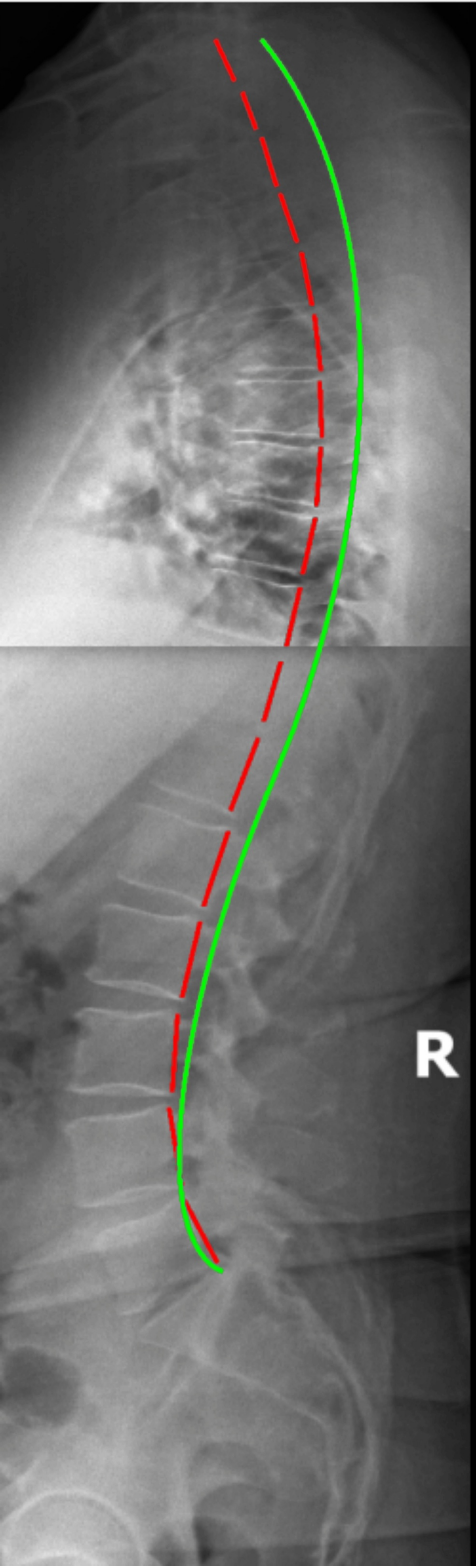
Three-year lateral thoracic and lateral lumbar radiographs demonstrating slight increased forward sagittal balance Long-term stitched lateral lumbar and lateral thoracic show some increase in positive translation of the torso; however, the patient maintained good contours with no in-office treatment for three years.
